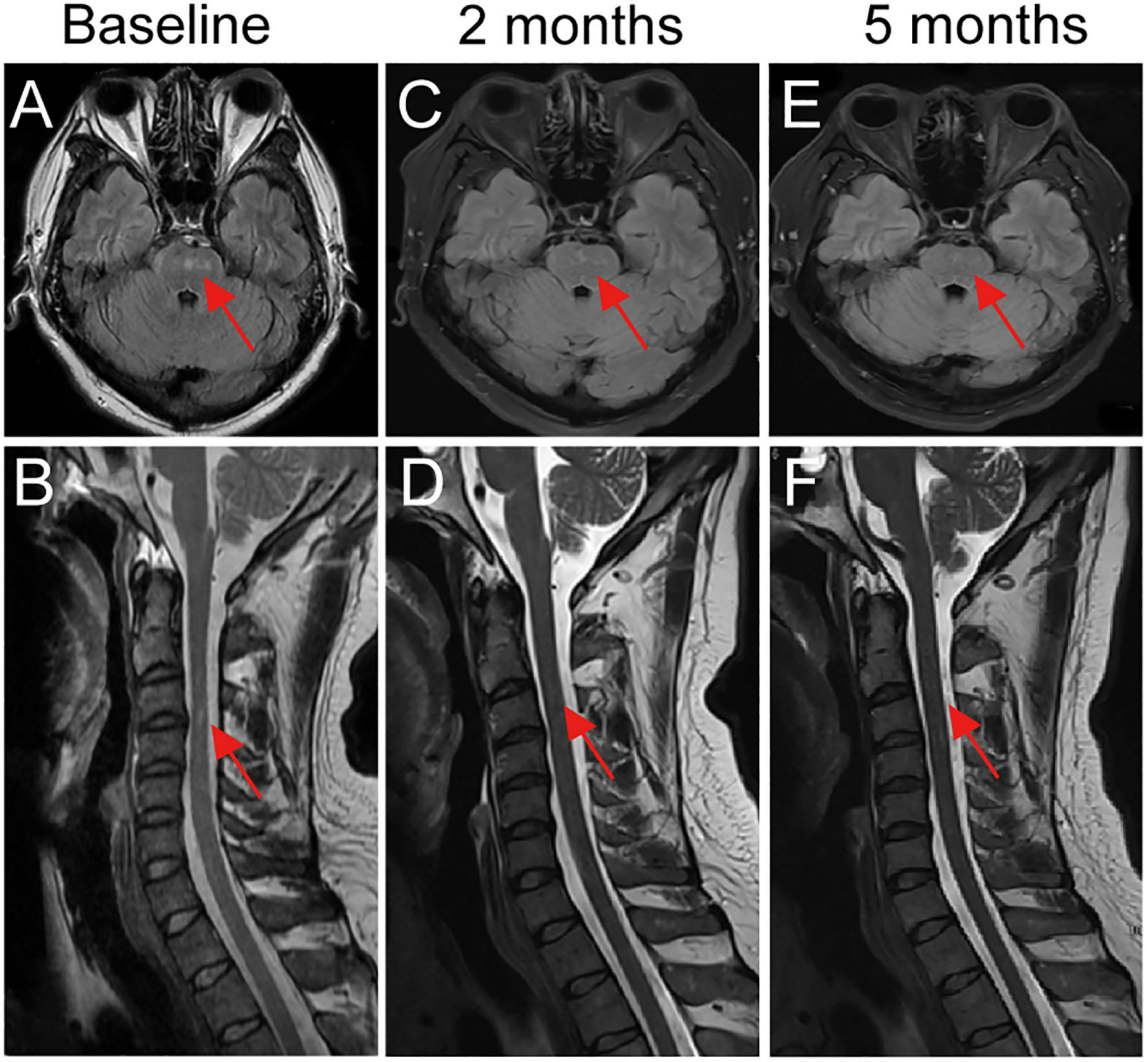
Longitudinal brain and spinal MRI changes in a patient with GFAP- A treated with telitacicept. (A, B) Baseline MRI revealed hyperintense lesions in the pons and cervicothoracic spinal cord. (C, D) After 2 months of telitacicept therapy, lesions showed marked reduction in size and signal intensity in both the brainstem and spinal cord. (E, F) At 5 months, complete resolution of brainstem and spinal cord lesions was observed, consistent with sustained radiological remission. Red arrows indicate lesion sites.
